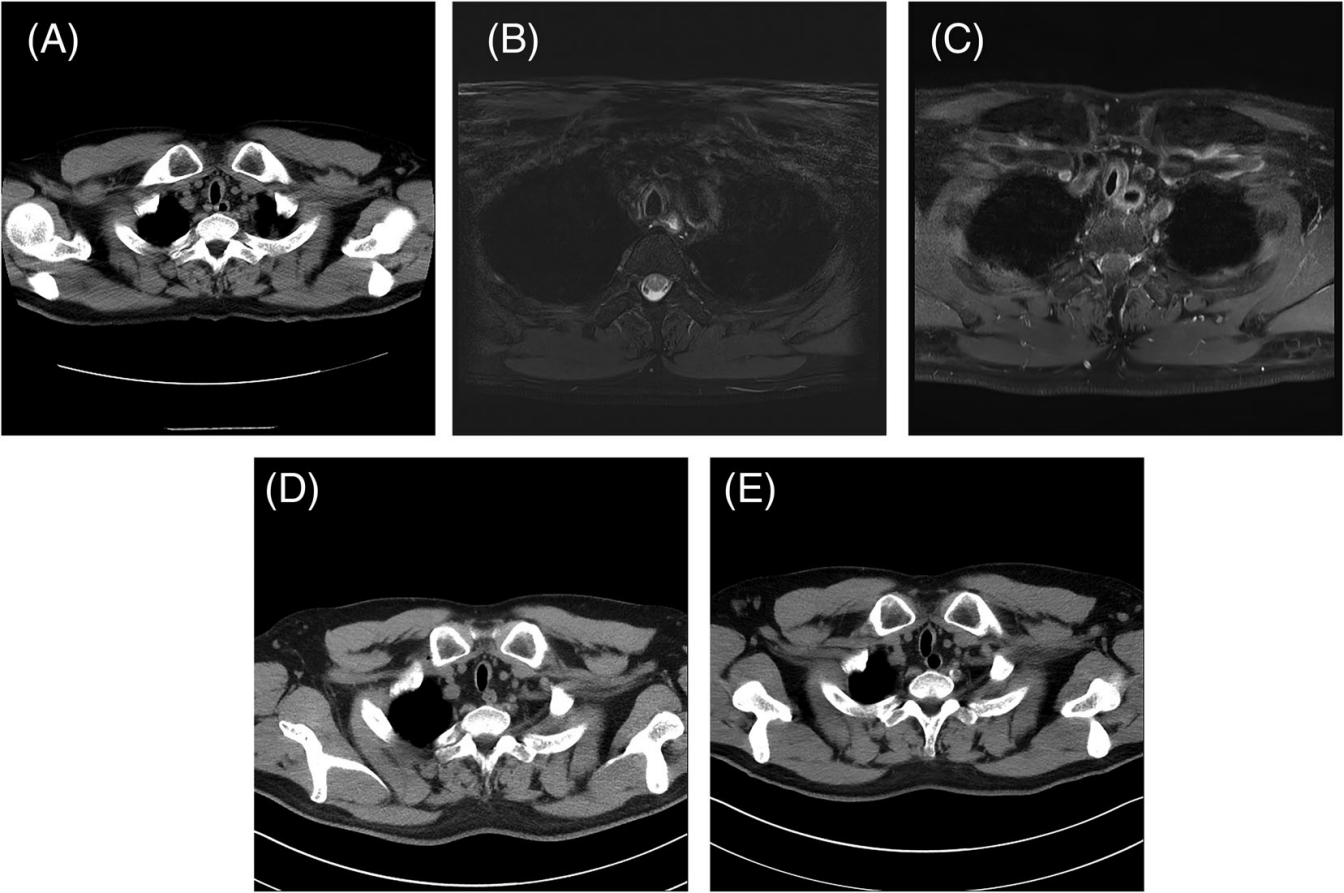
(A) Computed tomography (CT) of the chest revealed diffuse thickening of the cartilaginous portion of the trachea, with the posterior membrane spared. (B) Magnetic resonance imaging (MRI) of the chest showed that the periphery of the tracheal cartilaginous C‐ring exhibited hyperintensity on the T2‐weighted image and (C) enhancement on the post‐gadolinium T1‐weighted image. (D) Five months into treatment, follow‐up CT showed decreased thickness of the cartilaginous wall of the trachea, which remained stable at 10 months as shown in (E).
